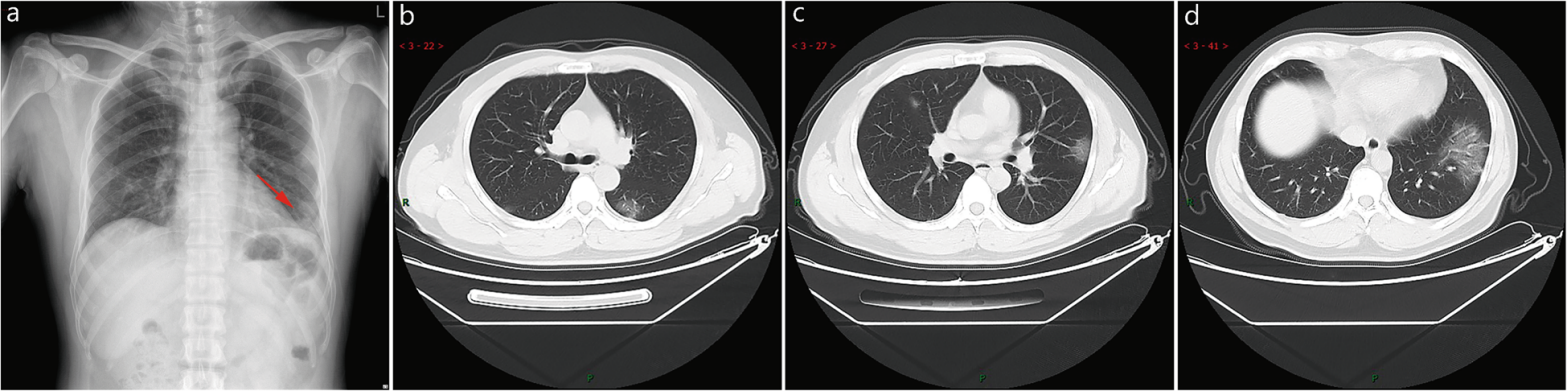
Typical CT / X-ray imaging manifestation (case 2). A 51 years old male with general muscle ache and fatigue for 1 week, fever for 1 day (39.1 ℃), anemia. Laboratory test: normal white blood cells (9.24 × 10^9^/L), lymphocytes percentage (5.1%), decreased lymphocytes (0.47 × 10^9^/ L), decreased eosinophil count (0 × 10^9^/L), increased C-reaction protein (170.91 mg/L), increased procalcitonin (0.45 ng/ml), increased erythrocyte sedimentation rate (48 mm/h). Imaging examination: a shows patchy shadows in the outer region of the left lower lobe, b shows large ground-glass opacity in the left lower lobe, and c shows subpleural patchy ground-glass opacity in posterior part of right upper lobe and lower tongue of left upper lobe, d shows large ground-glass opacity in the basal segment of the left lower lobe
